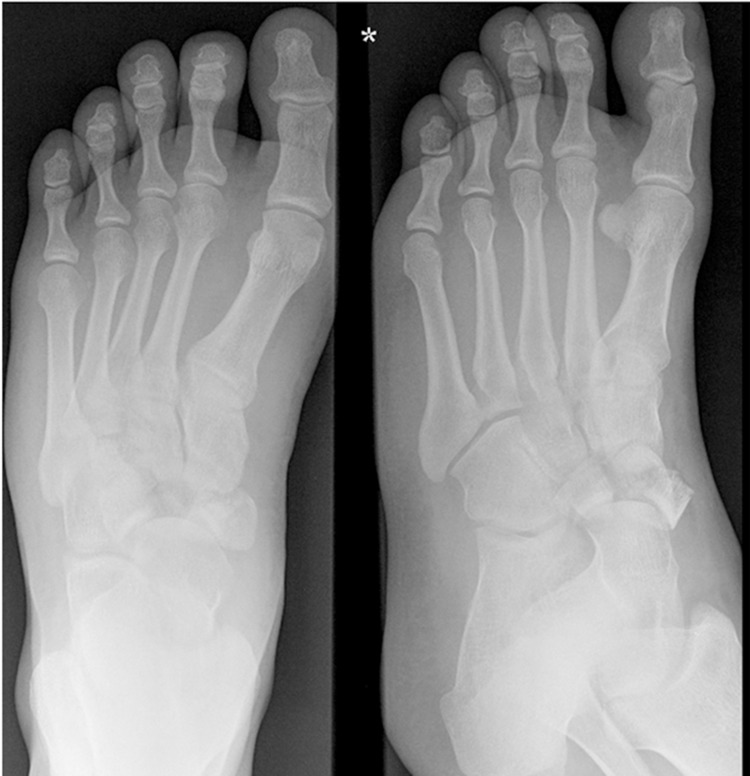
Pre-operative radiograph of a comminuted navicular fracture
